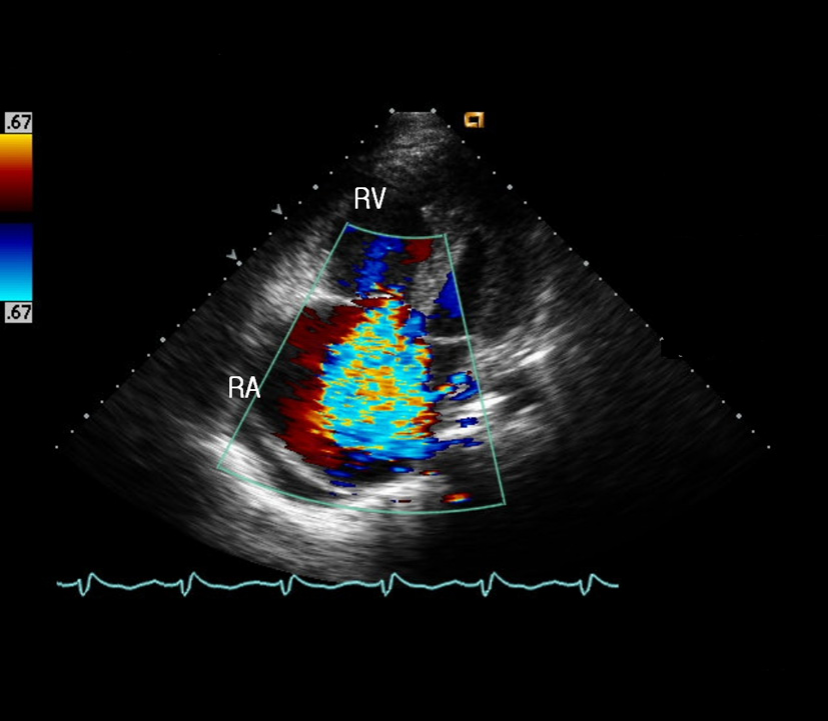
Transthoracic echocardiography at the apical 4 chamber view showed right ventricular and atrial dilatation and severe tricuspid valvular regurgitation. RA, right atrium; RV, right ventricle.
